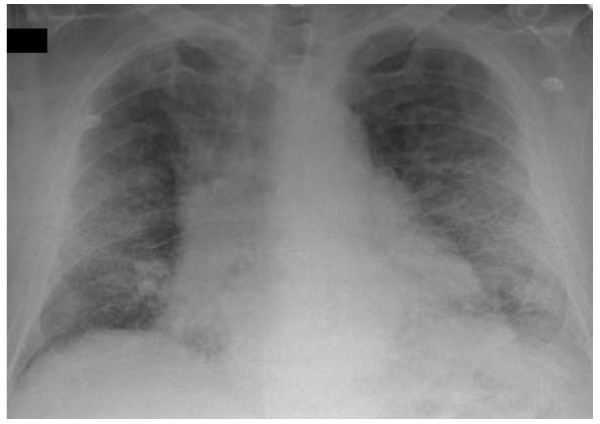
Chest radiograph showing bilateral interstitial reticular shadowing with loss of lung volume.
